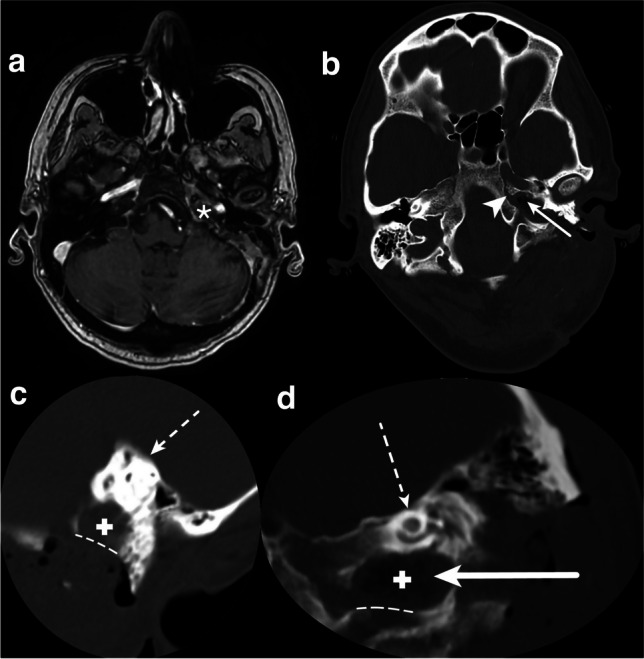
Postoperative cranial magnetic resonance imaging (MRI) and computed tomography (CT) confirming tumor removal via the TI-A a Postoperative axial MRI after gadolinium administration demonstrating no contrast-enhancing tumor mass (white star). Reformatted and tilted b axial, c coronal, and d sagittal cranial CT reveal access to the tumor cavity (white cross), located beneath the inferior part of the extradural petroclival junction (PJ) (white arrowhead), via an osseous infralabyrinthine approach pathway (white arrow). The jugular bulb covered by a thin shell of bone (white dotted line) and the preserved bony labyrinth (dotted white arrow) are visualized
